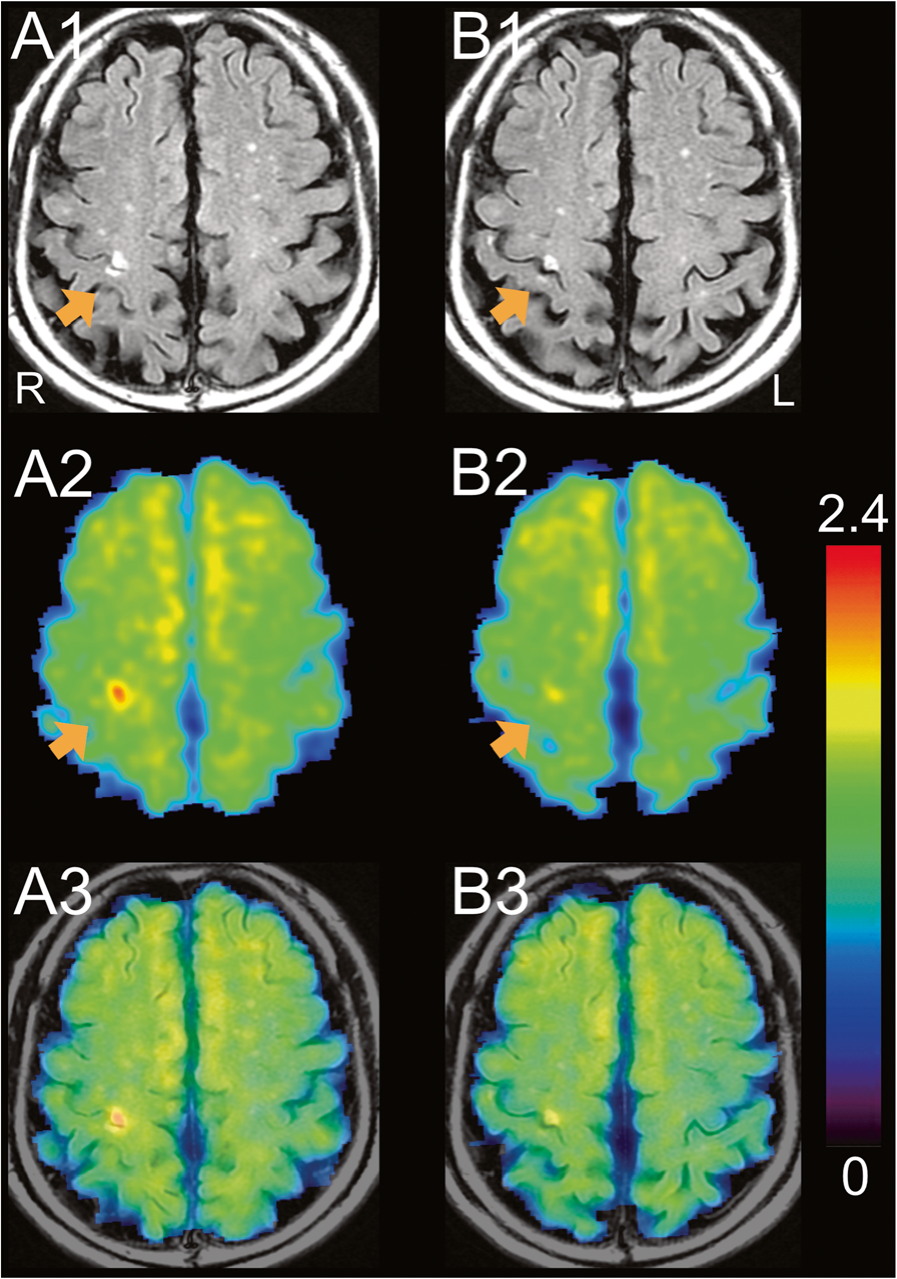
FLAIR and ^18^F-THK5351 PET images, 27 days (A) and 391 days (B) after stroke. FLAIR (A1, B1) and ^18^F-THK5351 PET (A2, B2) images are displayed in the upper and middle rows, respectively. The orange arrows represent the infarct lesion located around the right precentral and postcentral gyri. The fused images of FLAIR and ^18^F-THK5351 PET are displayed in the bottom row (A3, B3). The rainbow color scale represents the normalized uptake of ^18^F-THK5351, where cerebellar uptake was set as one
